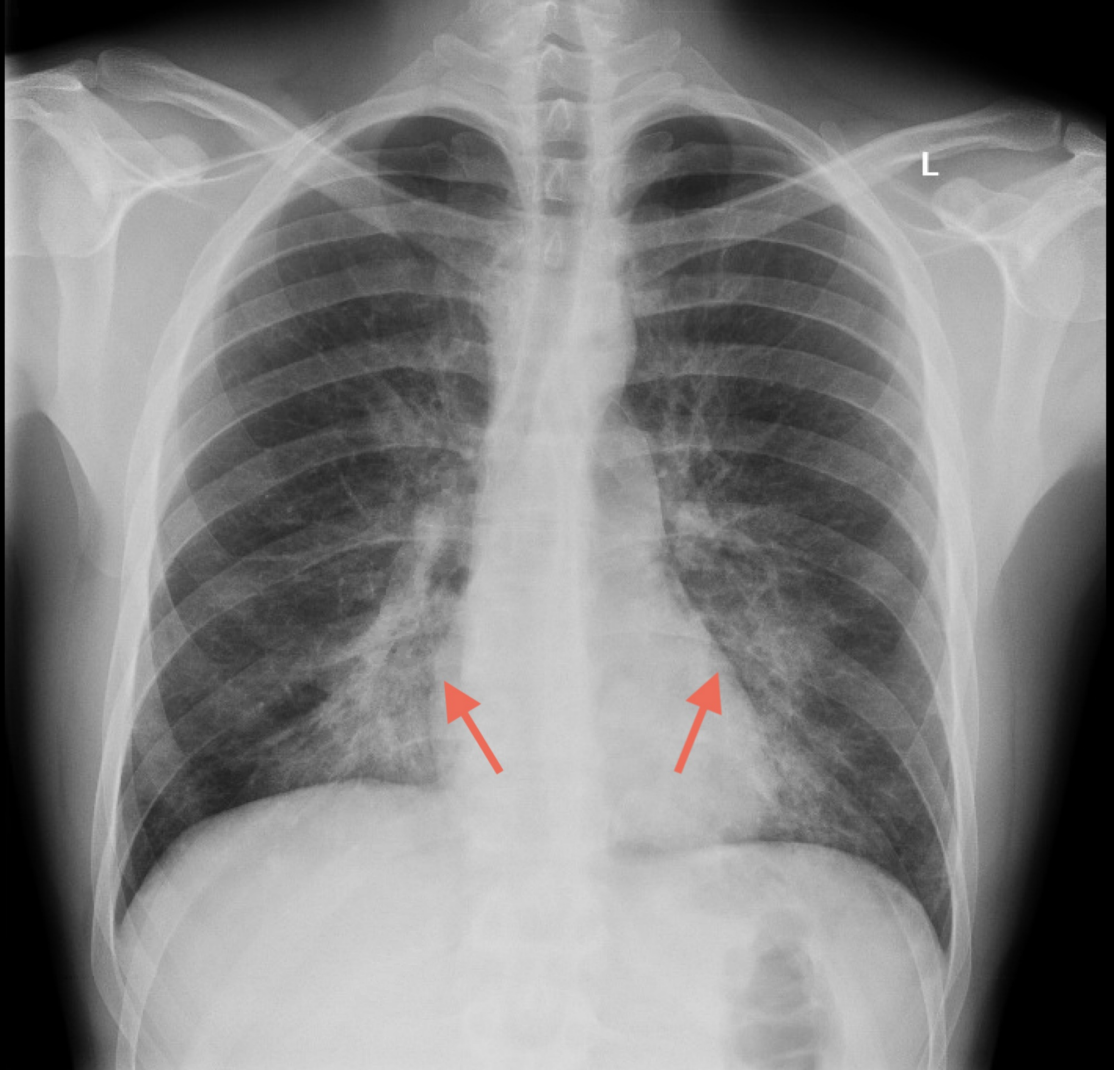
Chest x-ray (CXR) on the day of admission. Bilateral medial lung base infiltrates of uncertain chronicity were noted (red arrows).
